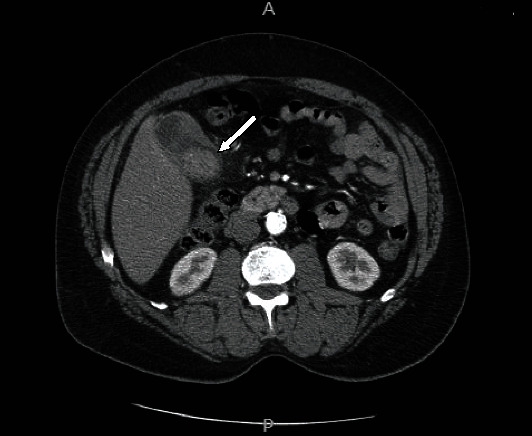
A noncontrast CT scan of the patient's abdomen revealing thickening of the gallbladder wall with a possible polyp (white arrow).
